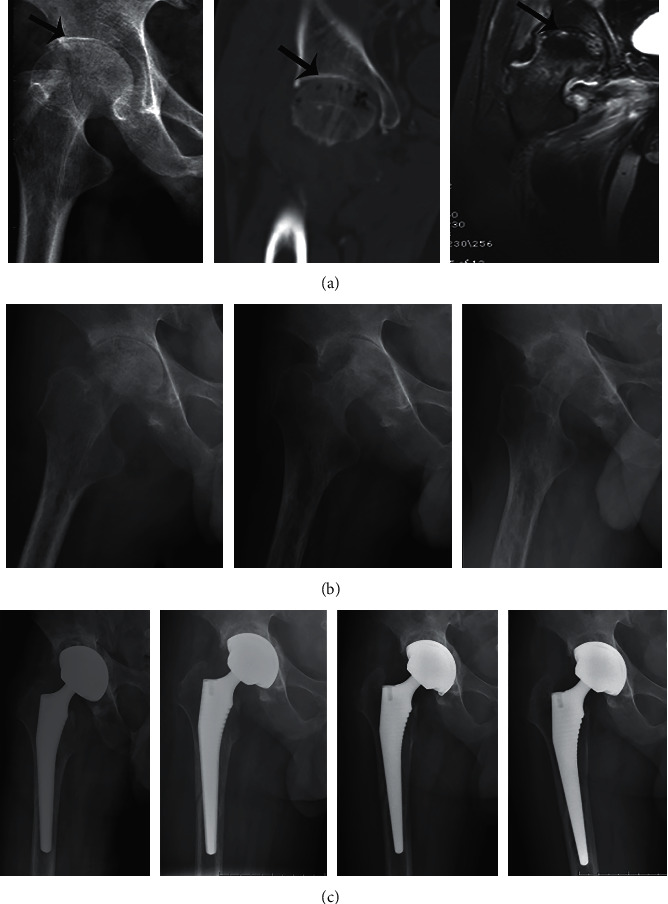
Radiographs of a 43-year-old man with pain in his right hip joint. (a) Anteroposterior X-ray and coronal CT images of the pelvis showed that the space of the hip joint became narrow and articular cartilage disappeared (arrow). Coronal MRI of the pelvis showed diffuse edema of the right femoral head. (b) X-ray examination of the hip joint at 4 months, 12 months, and 15 months after debridement operation. (c) The postoperative images of hip replacement at 7 days, 2 months, 5 months, and 15 months, respectively.
